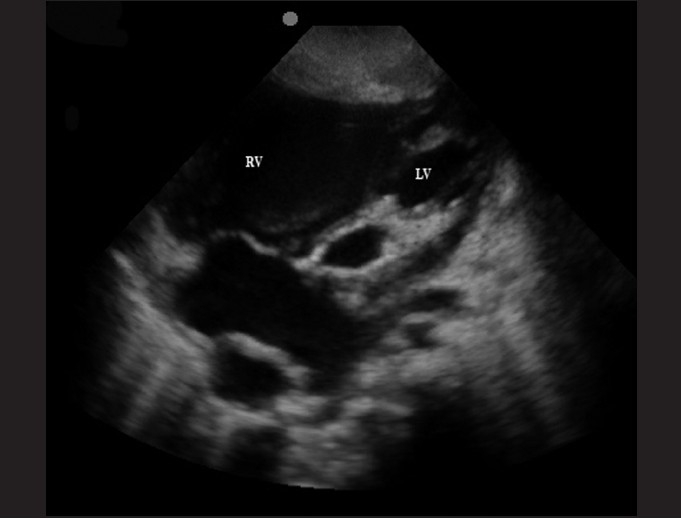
The apical 4-chamber view of a 5-year-old child with repaired Hypoplastic Left Heart Syndrome. Note the extremely small size of the left ventricle (LV) in relation to the right ventricle (RV)
